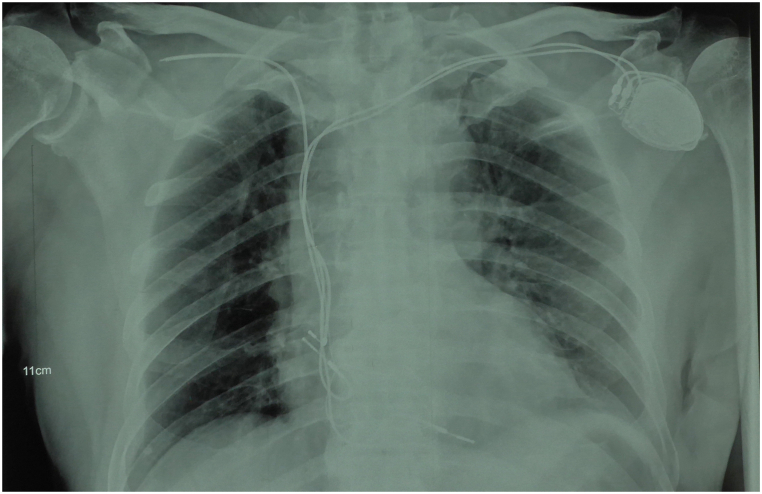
The Chest X-ray demonstrates the course of the residual pacemaker leads.
